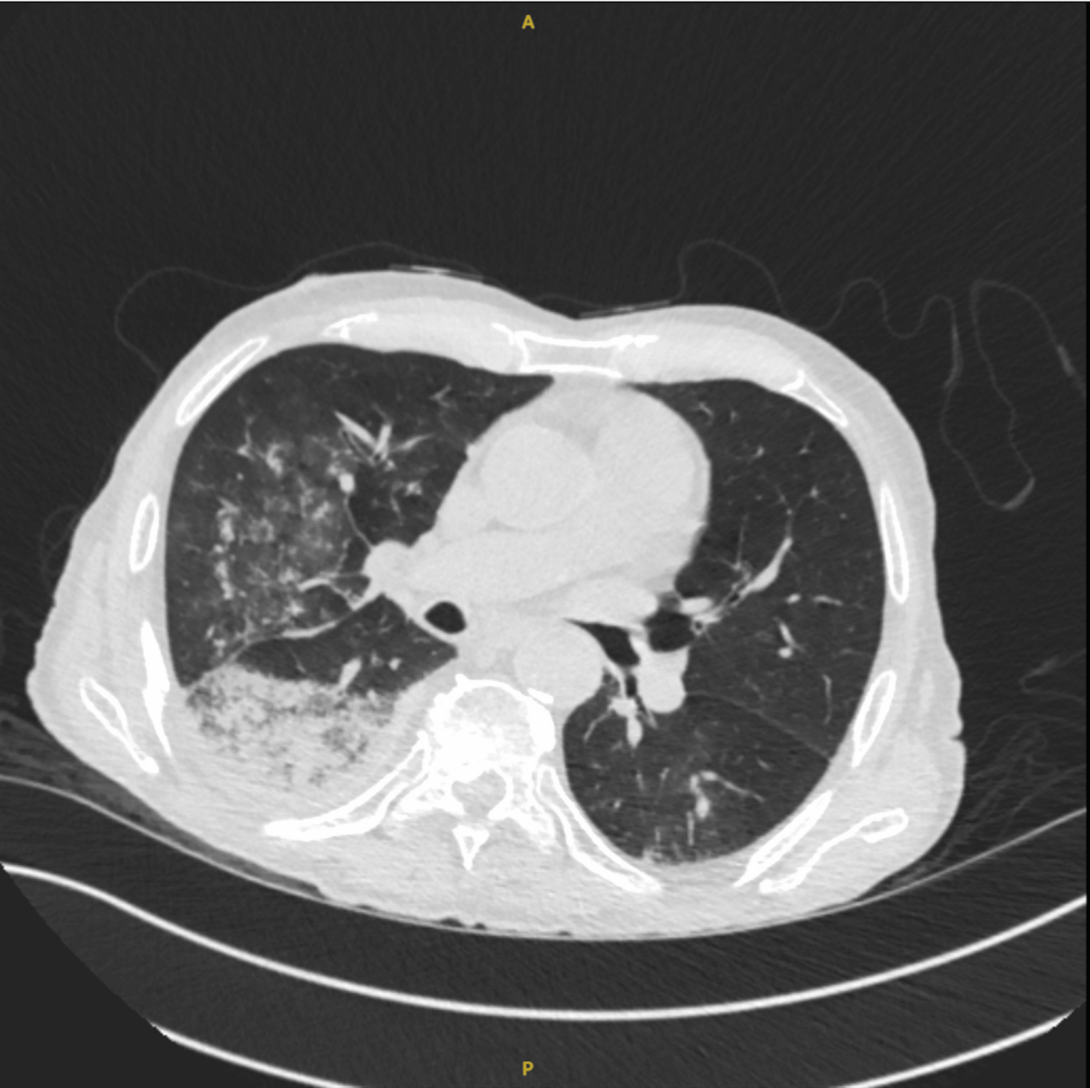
CT of the chest showing right lung parenchymal infective/inflammatory changes and confirming the absence of left-sided pneumothorax.
